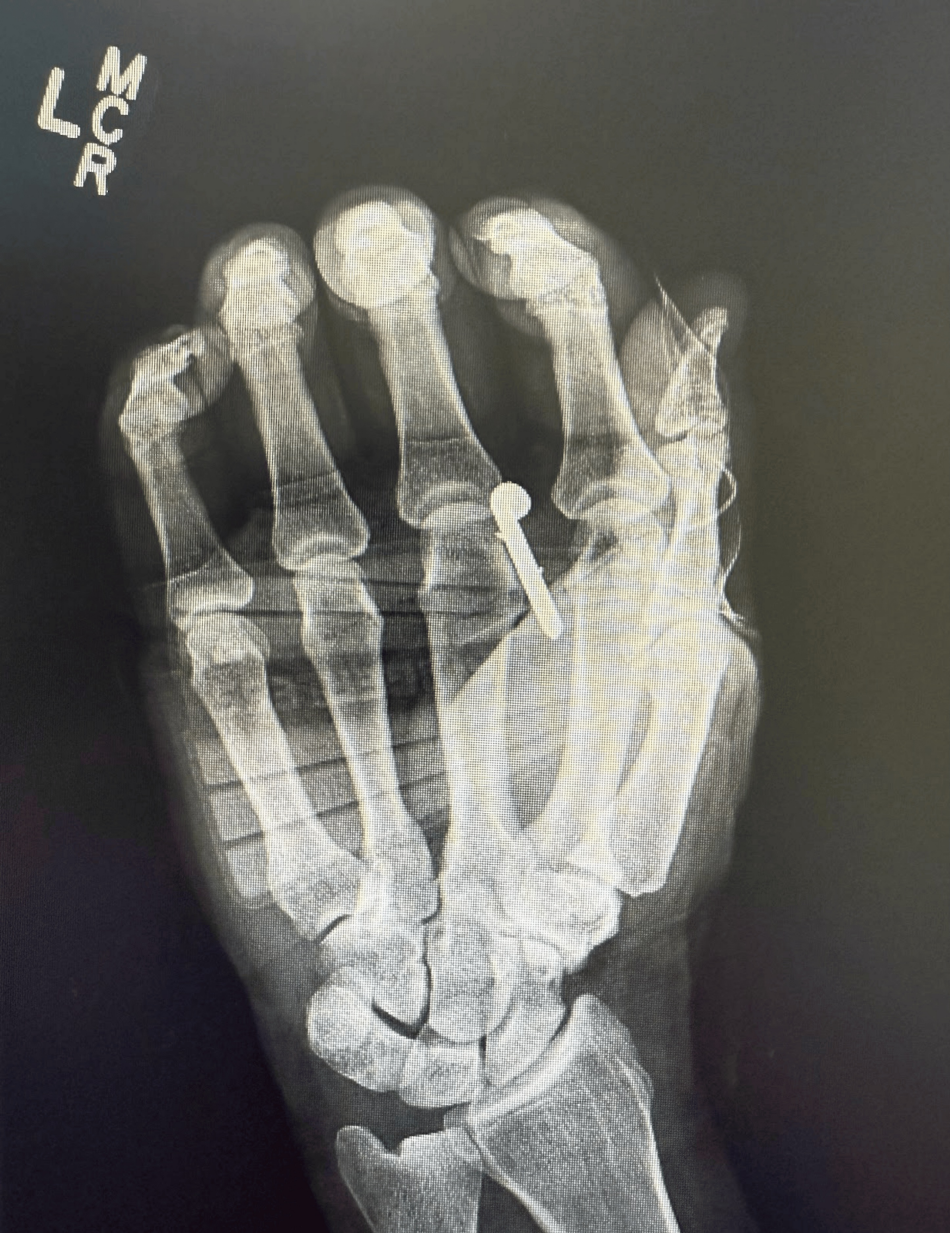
Anterior-posterior X-ray with nail position X-ray image showing the nail traversing between the 2nd and 3rd digits without evidence of associated bony injury.
